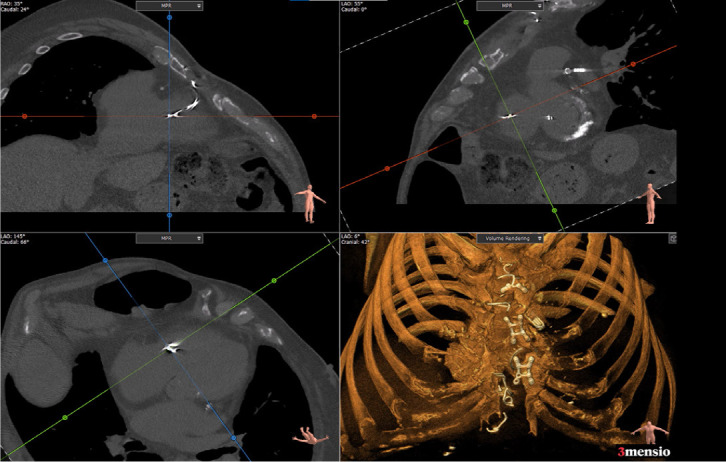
Multiplane non-contrast chest computed tomography: fragmented sternal
